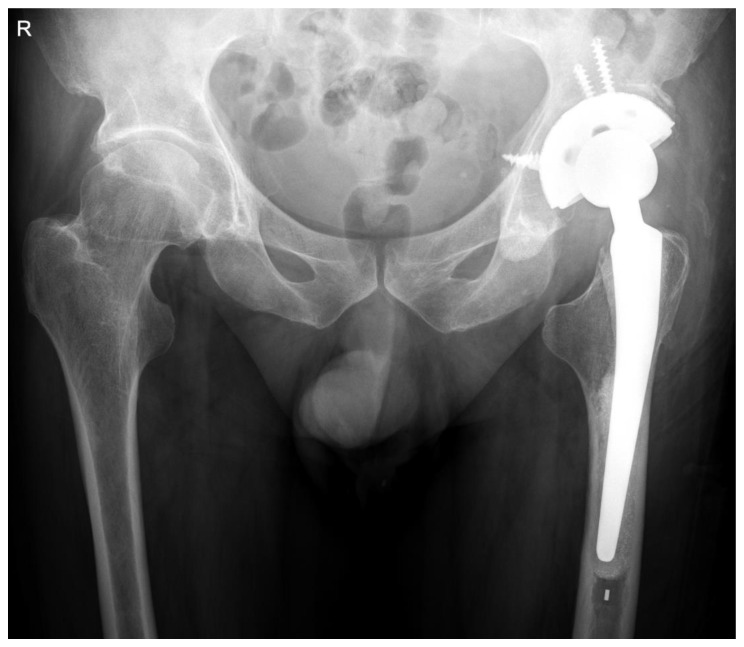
Postoperative radiographical aspect of the revision components.
